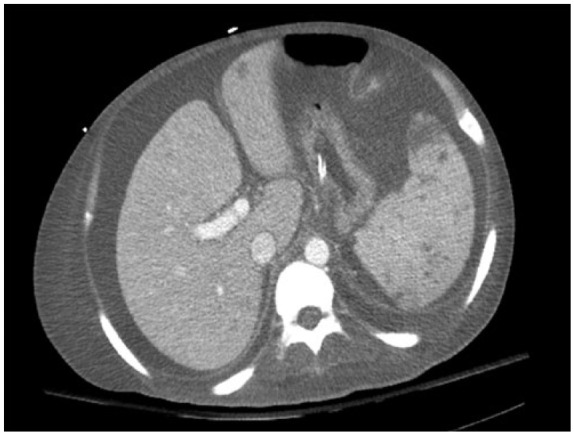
Abdominal computed tomography scan. Here splenomegaly, multiple hepatic and splenic lesions, and lymphadenopathy are seen.
